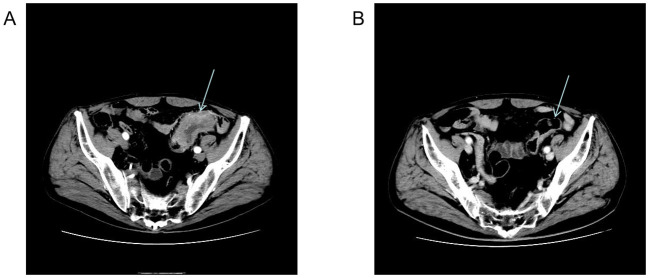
(A) CT demonstrated a space-occupying lesion in the left pelvic descending colon region; (B) CT imaging indicated a partial response according to the Response Evaluation Criteria In Solid Tumors(After two cycles of antitumor therapy).
